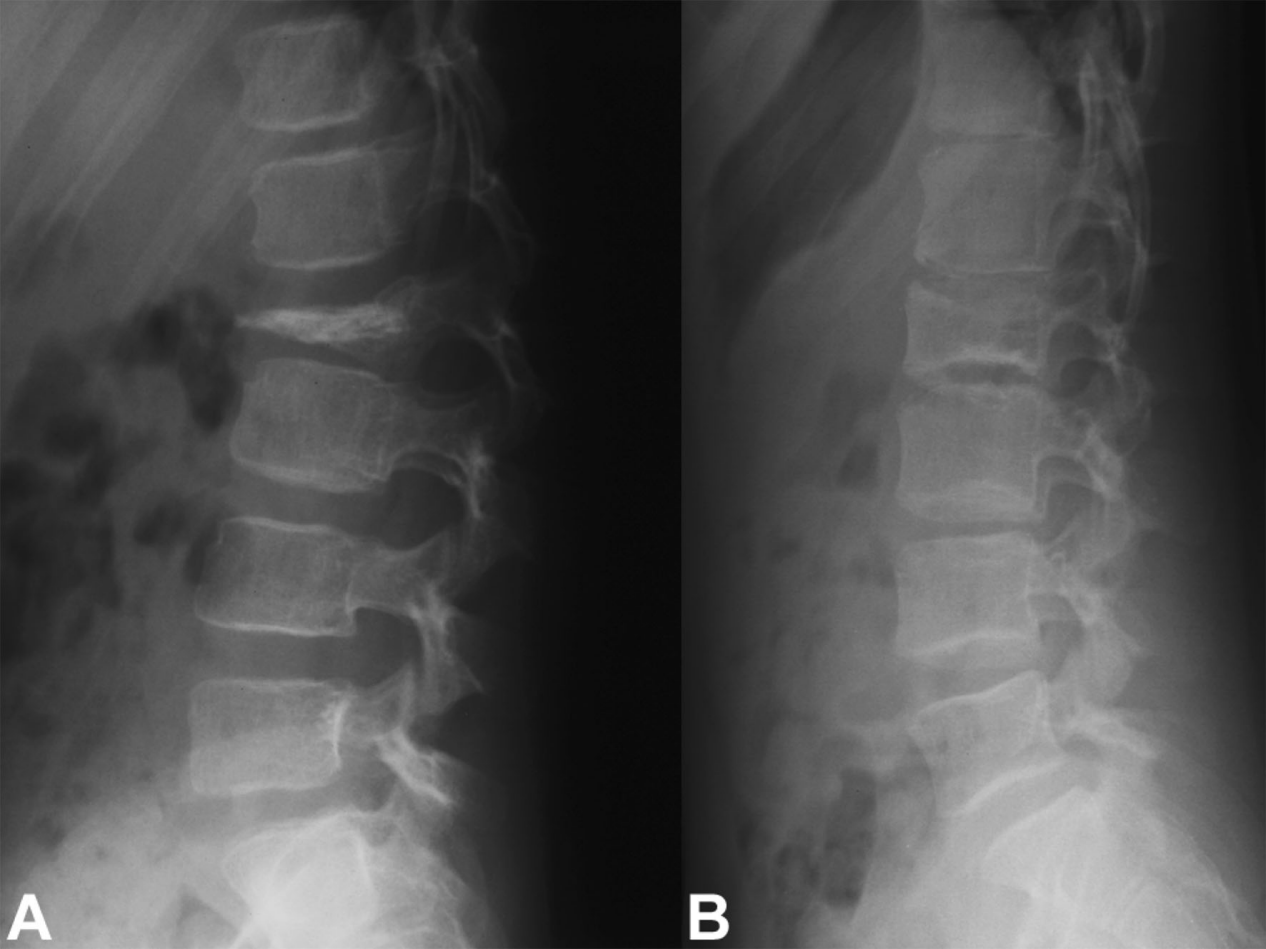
a Lateral radiograph of the lumbar spine of a 6-year-old girl with a painful osteolytic lesion of the L2 vertebral body with vertebral plana deformity. CT-guided frozen section biopsy showed eosinophilic granuloma; intralesional methylprednisolone injection was performed. b Lateral radiograph of the lumbar spine shows complete reconstitution of the lesion 7 years after diagnosis and treatment
